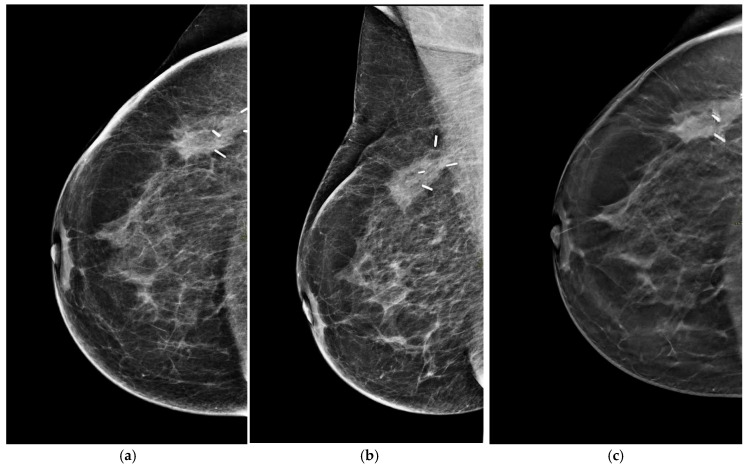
Six-month post-radiotherapy mammographic follow-up in a 56-year-old woman in axial (a) and oblique (b) projections, better visible in tomosynthesis. (c) shows skin thickening, breast edema, and increased density around the surgical clips, which corresponds on ultrasound to a fluid collection.
